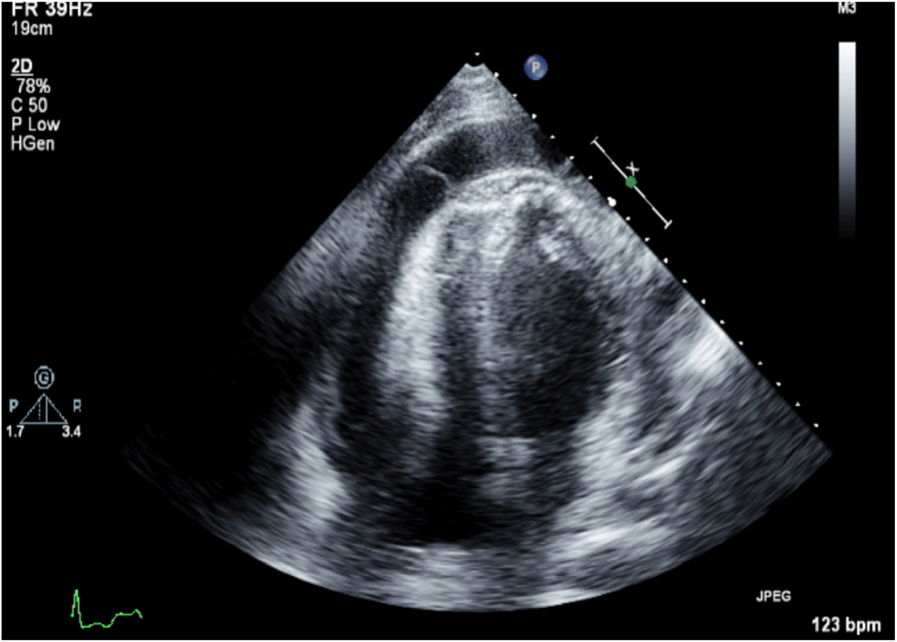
Transthoracic echocardiogram (four chamber view) showing pericardial effusion with presence of internal septations
